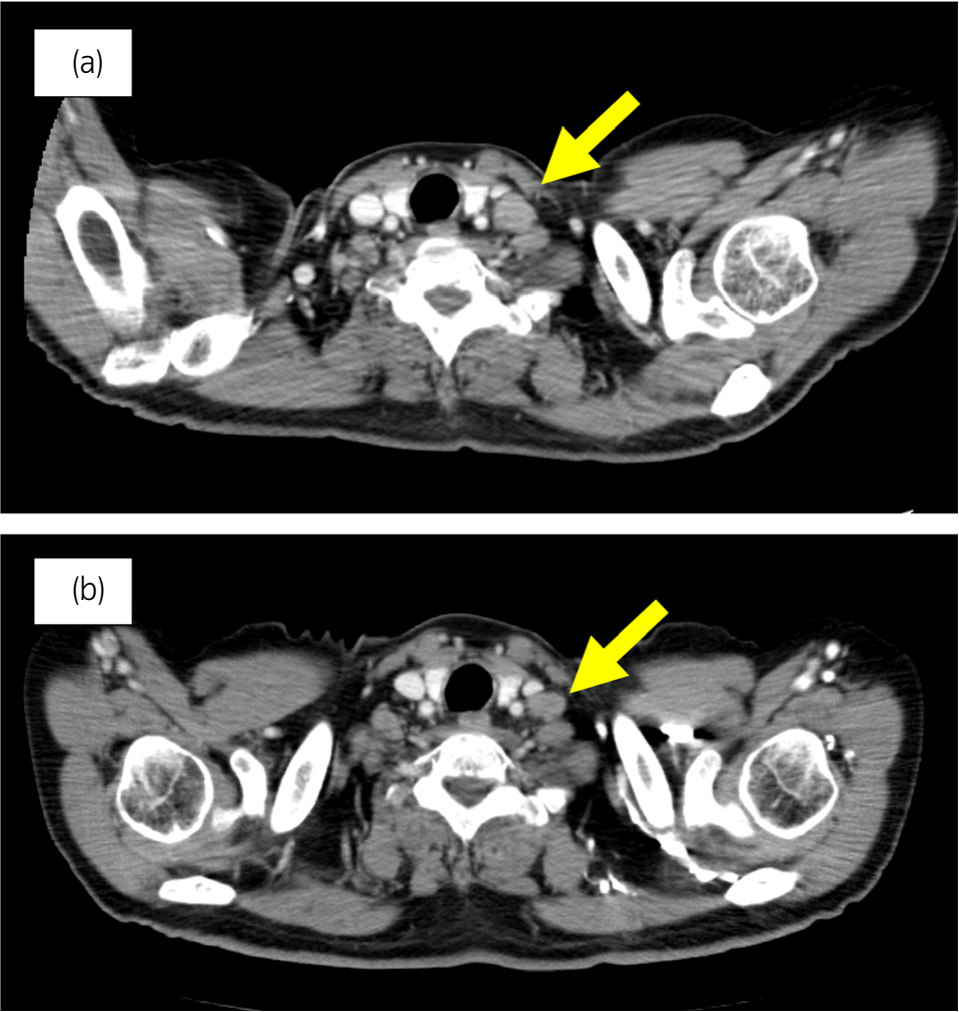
Case 1. CT images of the subclavian lymph node metastases before and 3 months after initiating olaparib. (a) Before treatment and (b) 3 months after olaparib treatment. Yellow arrow shows metastatic lymph node.
